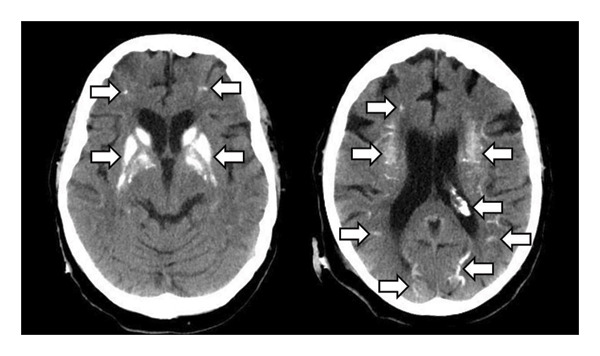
Cranial CT scan (transversal plane). Pronounced cerebral calcifications (arrows) in both basal ganglia, both cerebral hemispheres and choroid plexus in patient 2.
